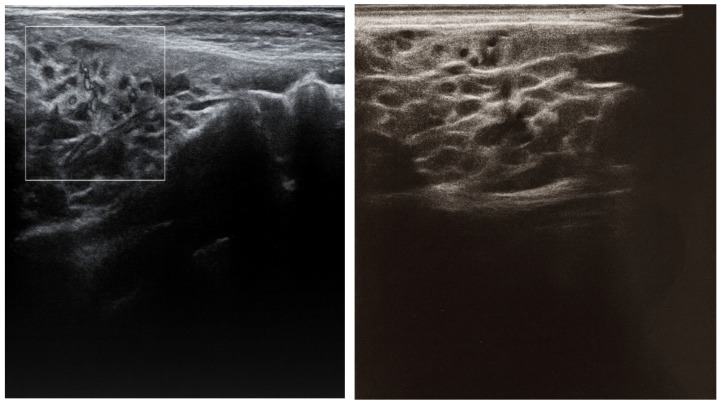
Submandibular gland ultrasound frames showing active inflammatory changes such as heterogeneous echotexture (“leopard-skin” or “snowstorm” appearance) and small hypoechoic foci, consistent with autoimmune sialadenitis.
